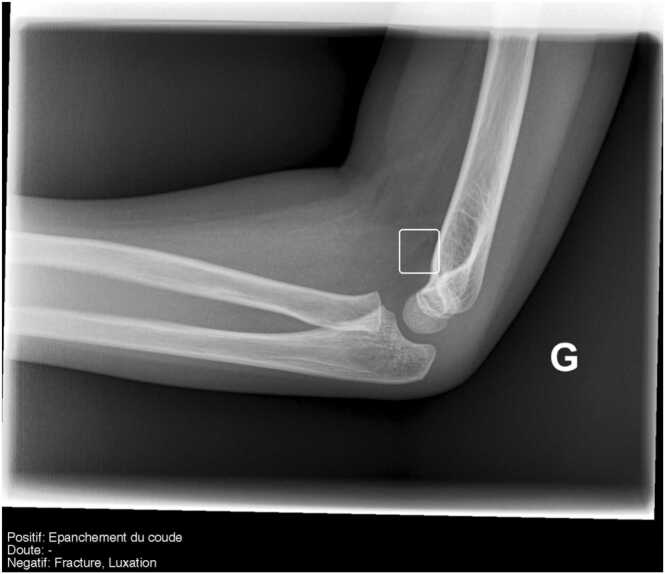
Example of an MSK discordant case correctly diagnosed by AI. Lateral view of an elbow radiograph in a child, reported as normal by the ED. The radiologist reported a joint effusion without visible fracture. AI output highlights the anterior elbow fat pad as the sign of the joint effusion. French translation: doute = doubt; luxation = dislocation; negatif = negative; positif = positive.
